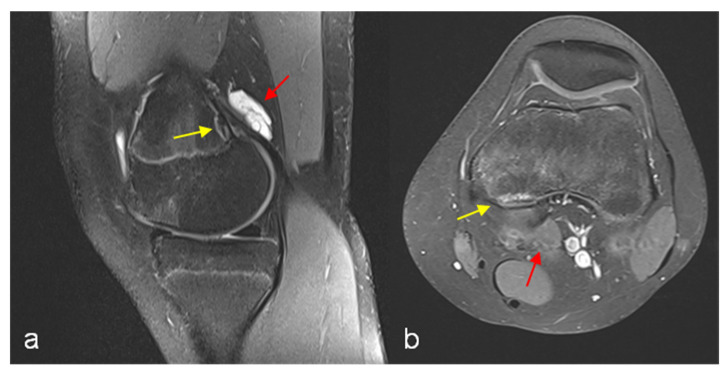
MRI left knee PD TSE FS sagittal reconstruction (a) and T1 TSE FS (Gd contrast agent) transversal reconstruction (b). Cystic fluid-filled lesion above the femoral origin of the medial gastrocnemius head with marginal Gd enhancement (red arrows), discrete signal enhancement at the tendinous insertion site. Cortical irregularity (DFCI) with signal enhancement and low-signal marginal sclerosis (yellow arrows).
